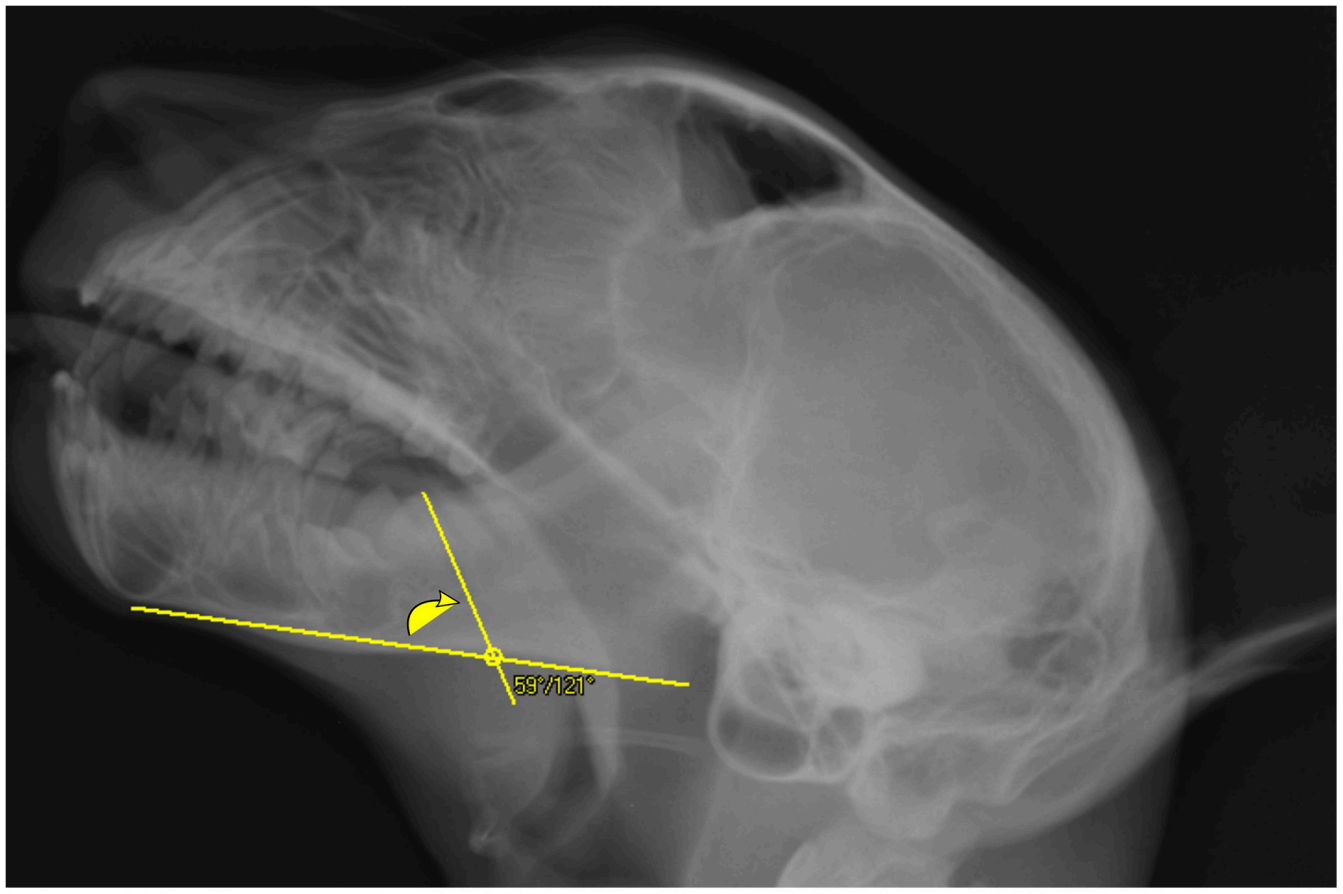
Depiction of a line drawn through the caudal cusp and apex of the molar tooth crosses the mandibular length2 line on the lateral skull radiograph of a cheetah cub. The inside angle where these two lines cross was measured. On this cropped image the 100 mm scale bar is not visible.
